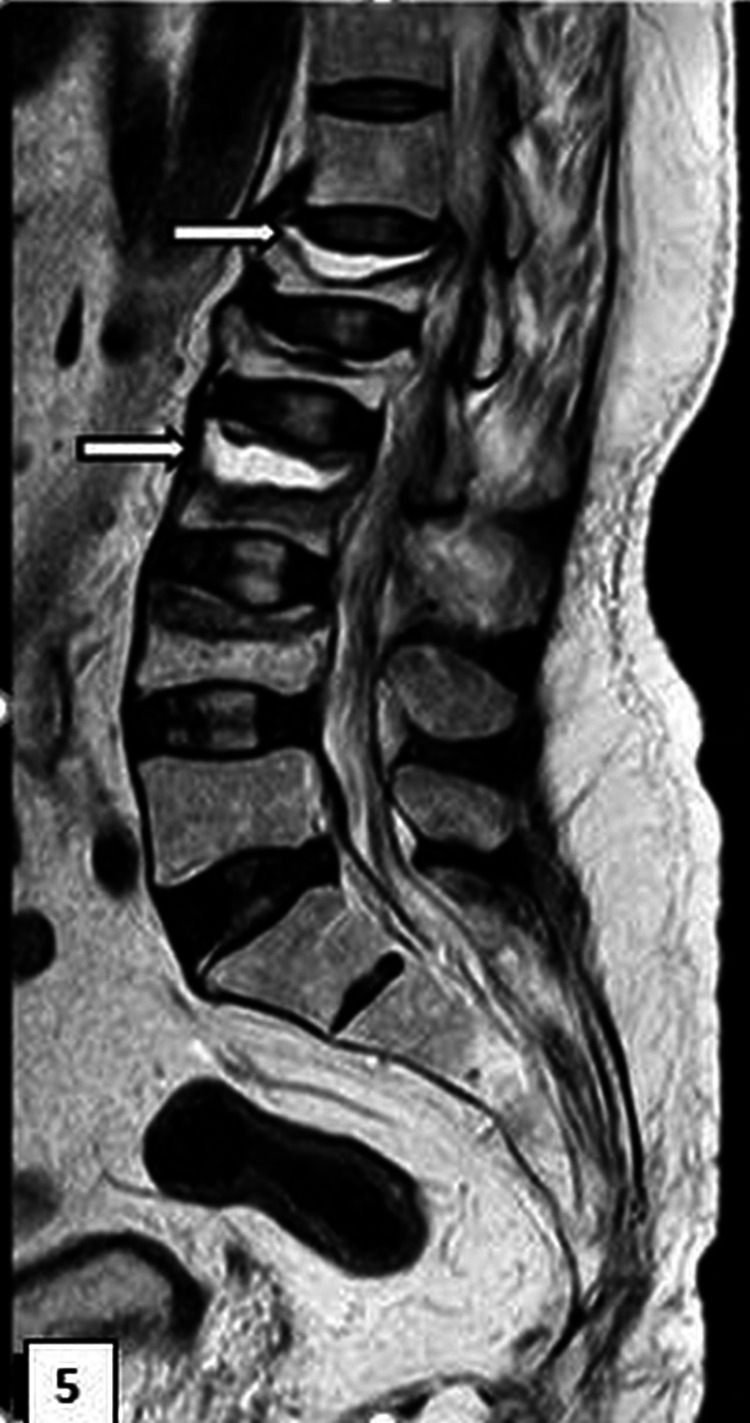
Magnetic resonance image of the lumbosacral spine (sagittal T2-weighted image) A fluid cleft (arrow) below the superior endplates of L1 and L3, indicative of Kummell’s disease, is shown. L: lumbar.
